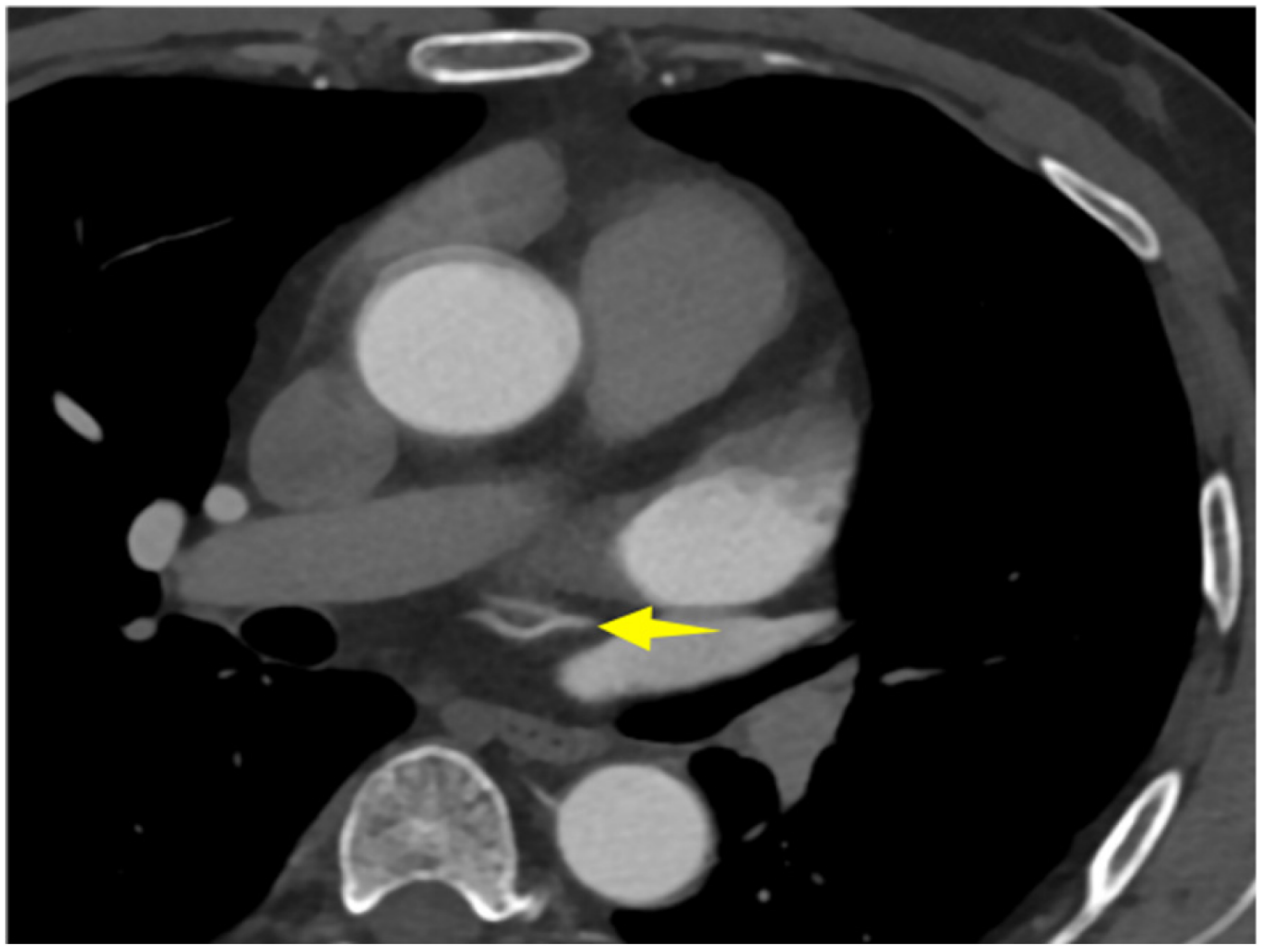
Coronary CTA showed a feeding artery of myxoma from LCX.
